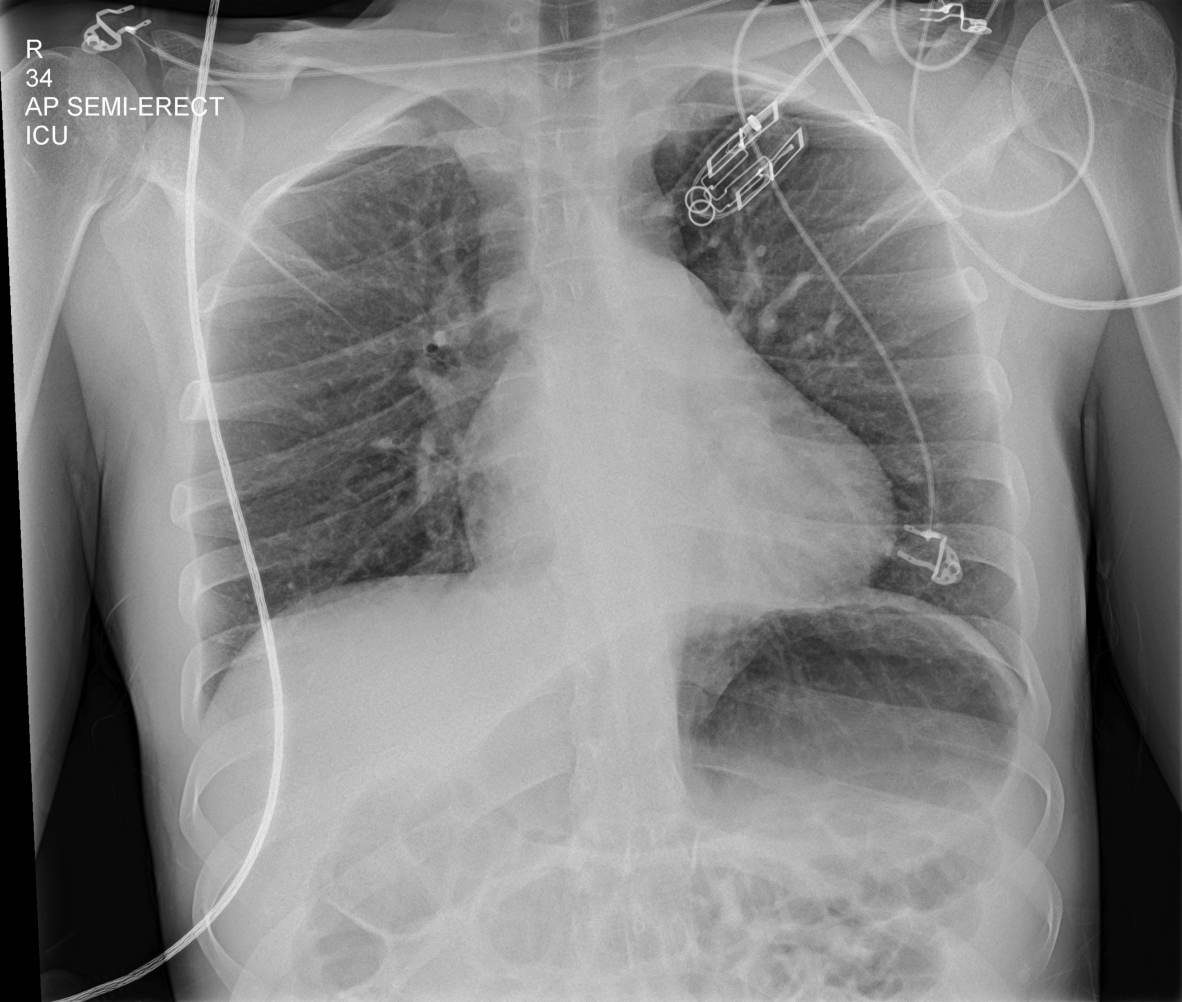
Chest X-ray showing clear lung fields
